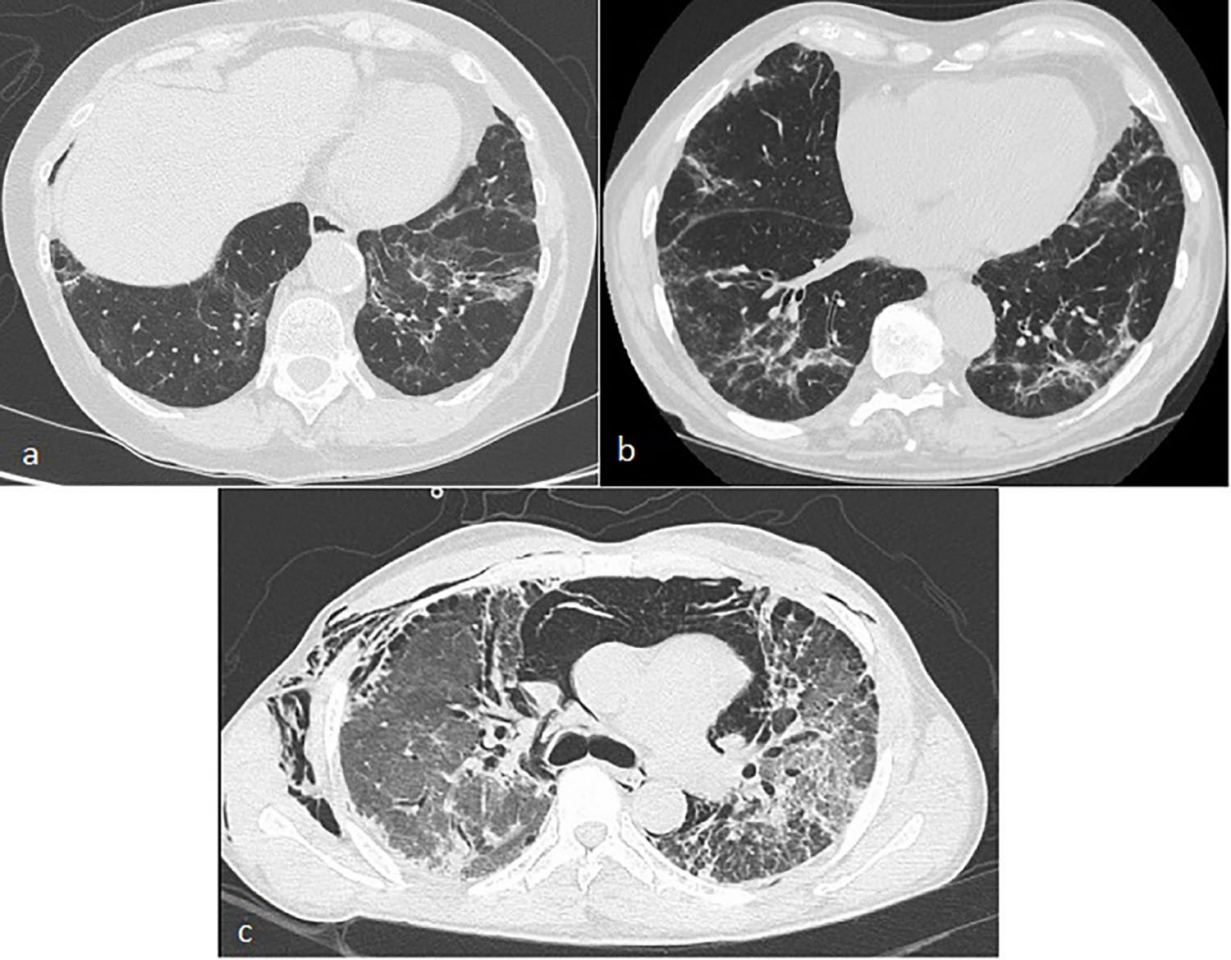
HRCT images. a: Fibrosing OP in a patient with dermatomyositis; b: NSIP pattern in a patient with anti-synthetase syndrome (Anti-Jo1 +); c: rapidly progressive ILD complicated by pneumomediastinum and subcutaneous emphysema in a patient with Clinically Amyopathic Dermatomyositis and anti-MDA5-antibodies positivity
